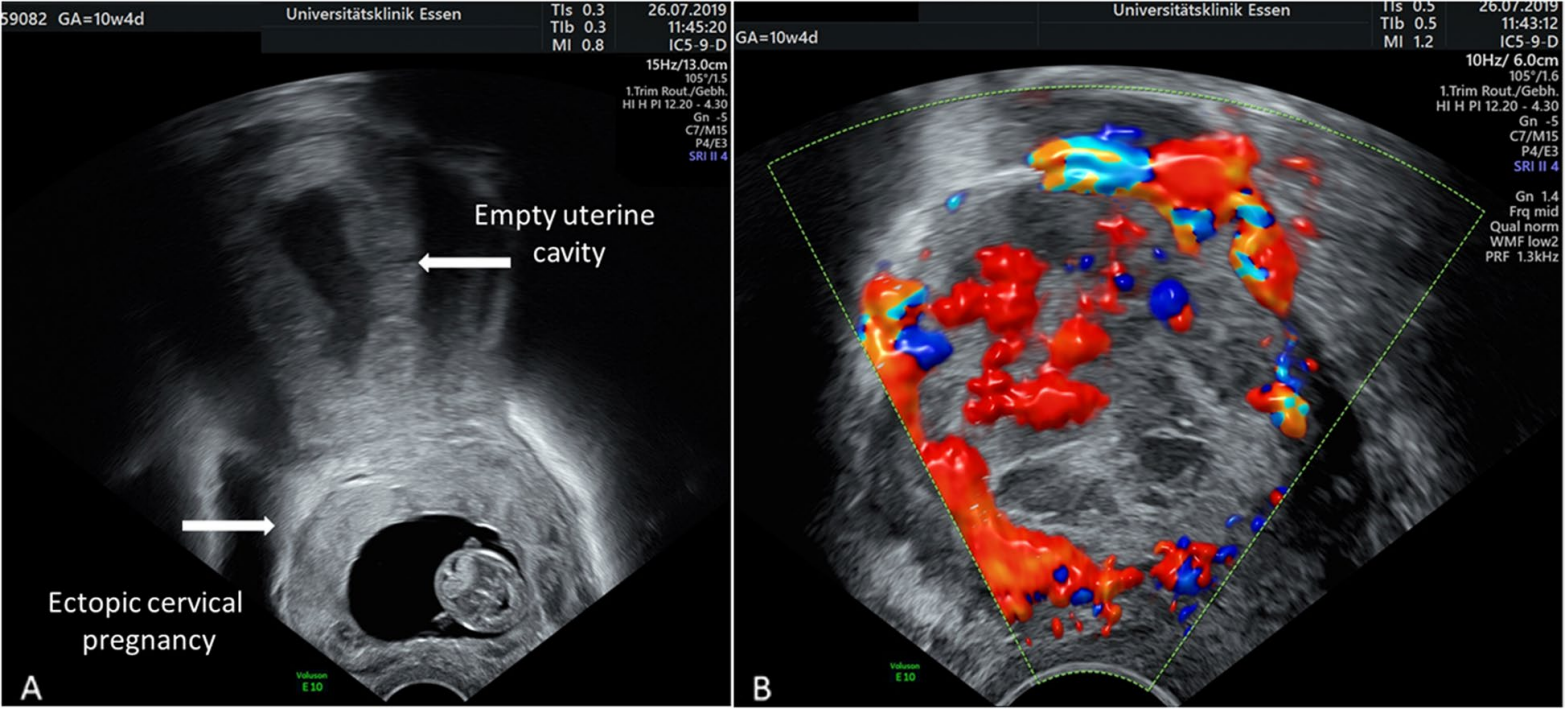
A Cervical ectopic pregnancy with the gestational sac was located between the internal and external cervical os. B Strong vascularization and lacunae in vaginal Doppler sonography
